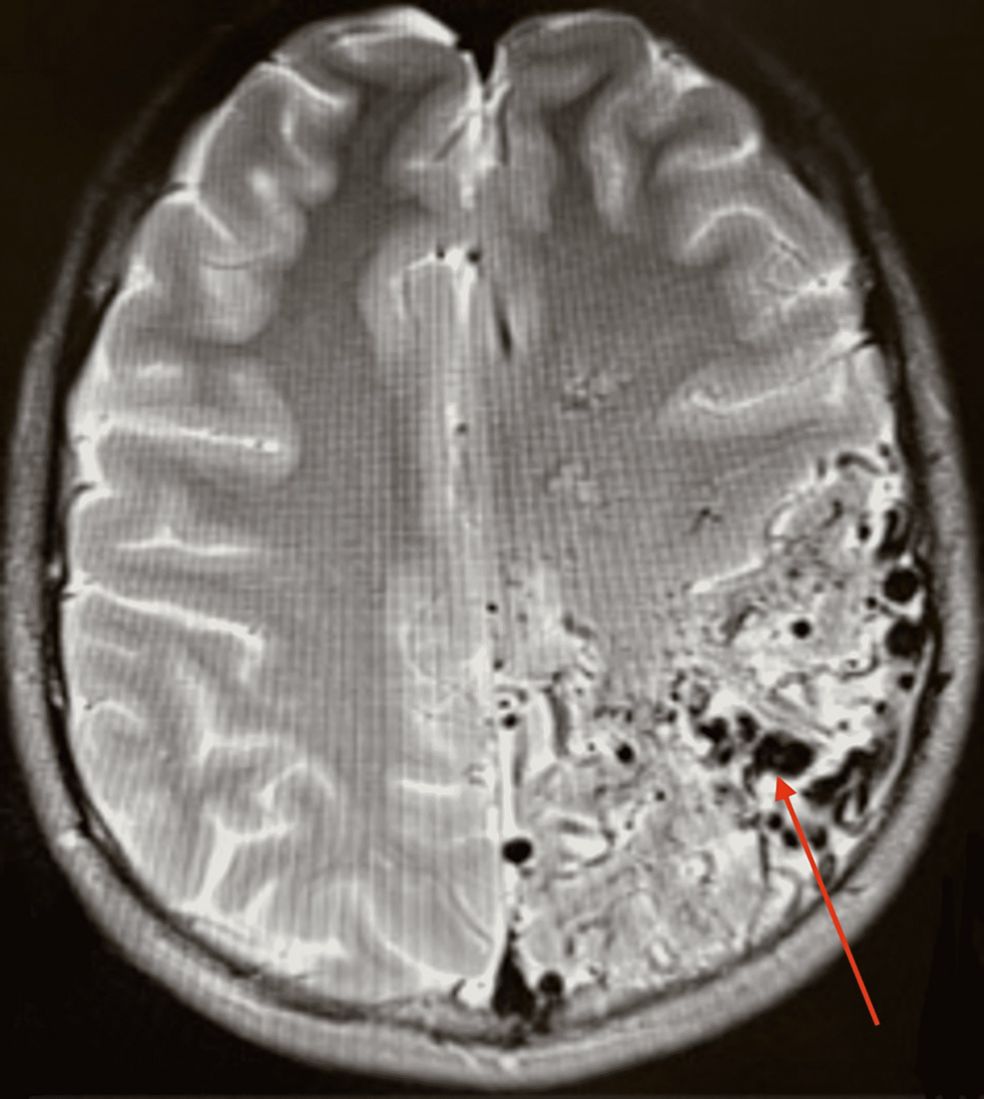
T2-weighted MRI This figure shows an AVM in the frontoparietal region with several flow voids and a characteristic “bag of worms” appearance (red arrow).
